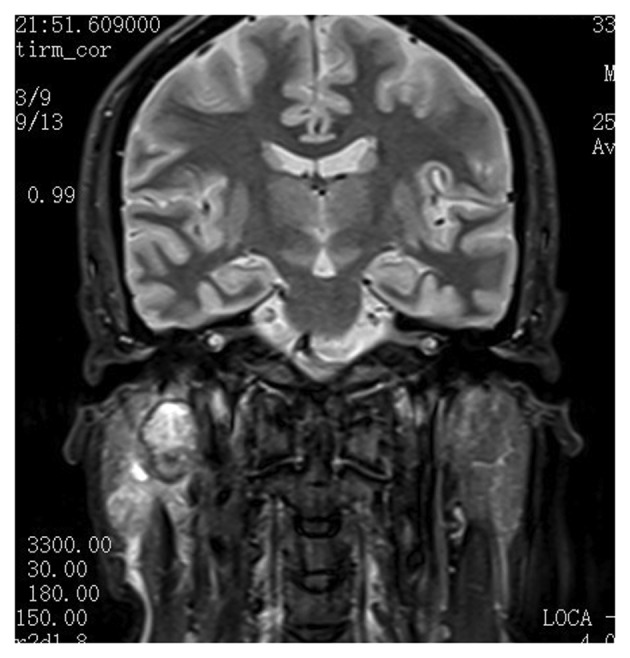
T1-weighted gadolinium enhanced magnetic resonance imaging revealing a tumor signal in the deep lobe of the parotid gland.
